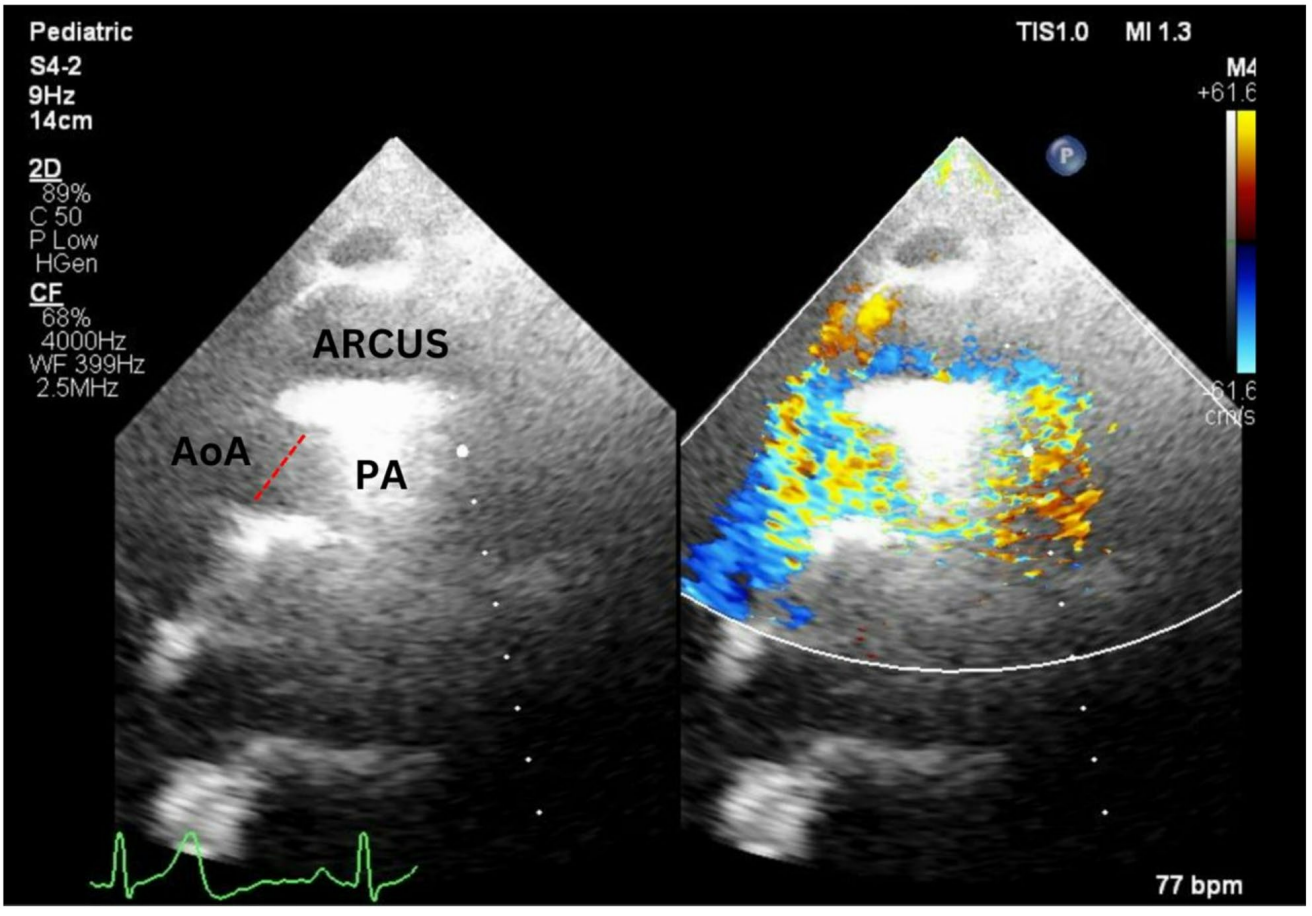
Pre-operative transthoracic echocardiogram demonstrating a large aortopulmonary window (red dashed line) with continuous left-to-right turbulent flow from the ascending aorta (AoA) to the main pulmonary artery (PA) on color Doppler imaging
